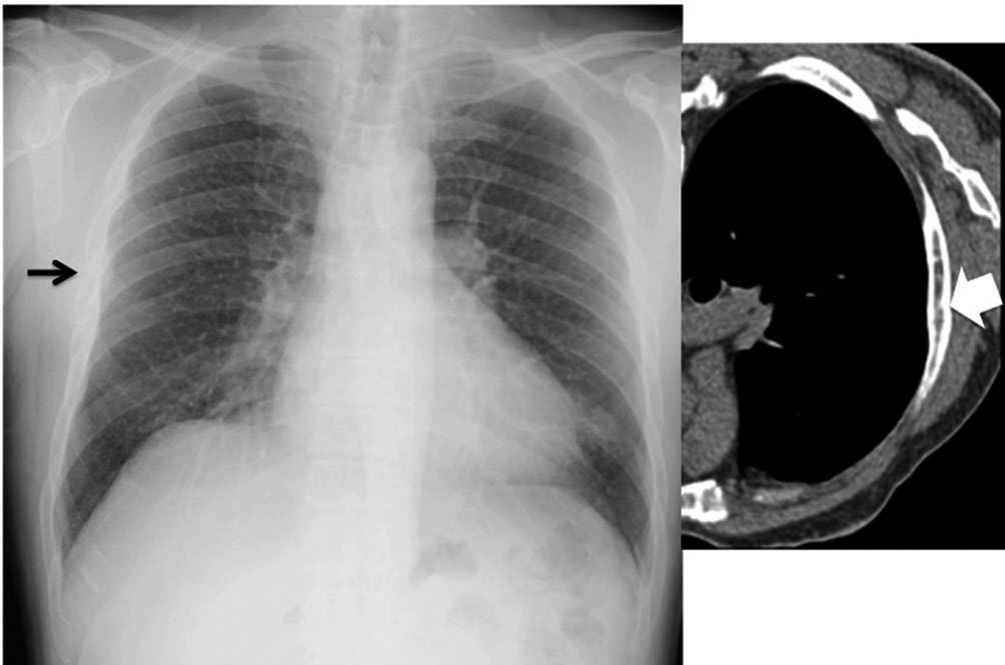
Discrepancy between chest radiography and thin‐slice CT reading results (Case 3). CT‐proven fractured rib (white arrow) misclassified as pleural plaques by three AIR Pneumo readers. A NIOSH B reader correctly classified this (black arrow) as fractured rib
